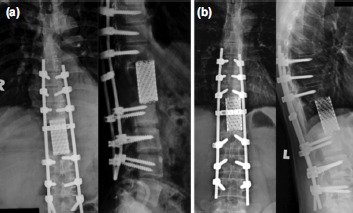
(a) Immediate post-operative radiograph. (b) One year post-operative radiograph showing no loosening of implants.
